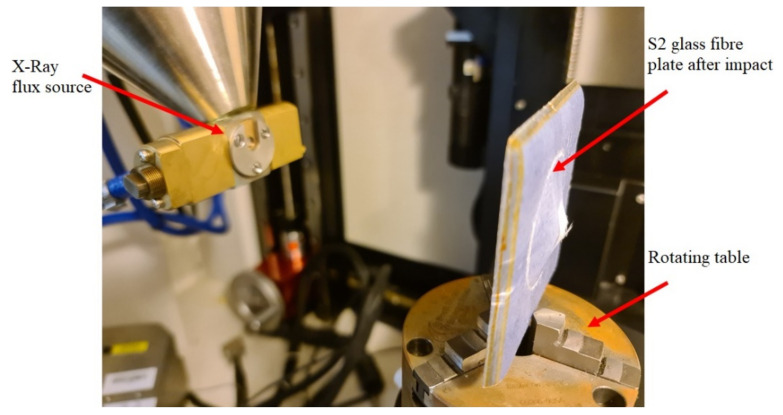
CT scan of glass fibre plates after impact.
